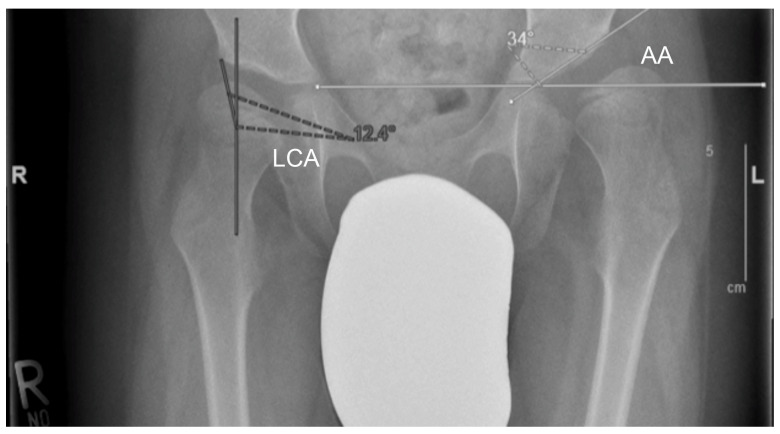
Anteroposterior radiographs of the pelvis were used to measure the lateral centre-edge-angle (LCA) and the acetabular angle of Hilgenreiner (AA). To measure the LCA, a best fitting circle inside the femoral head was drawn. The angle was then measured between two lines from the centre of this circle, one vertically and the other one to the lateral acetabular edge. The AA was measured by using the angle between the Hilgenreiner’s line (line through both triradiate cartilage) and the acetabular roof.
